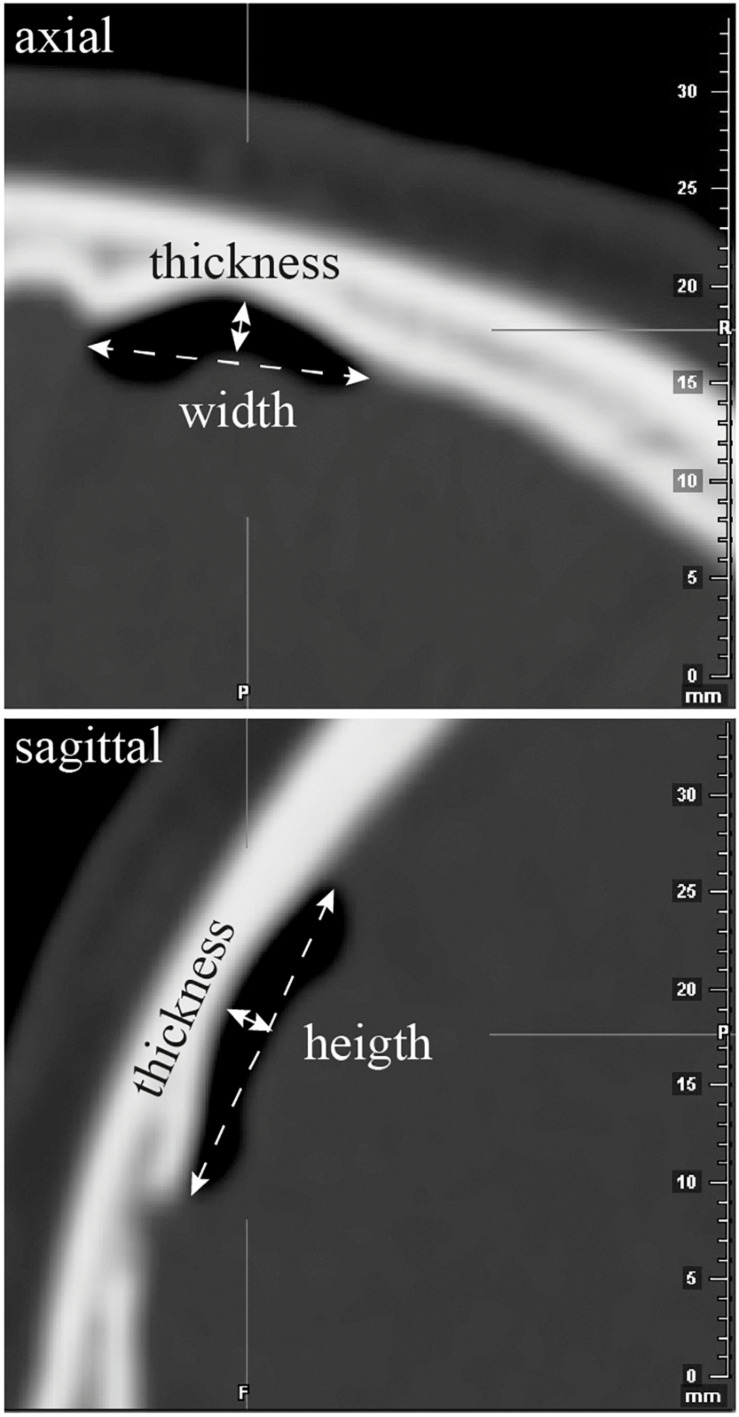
Air bubble visible against the frontal pole on postoperative CT scan. Principle of the measurements of the width, thickness, and height of the bubble air on axial and sagittal CT-scan slices.
